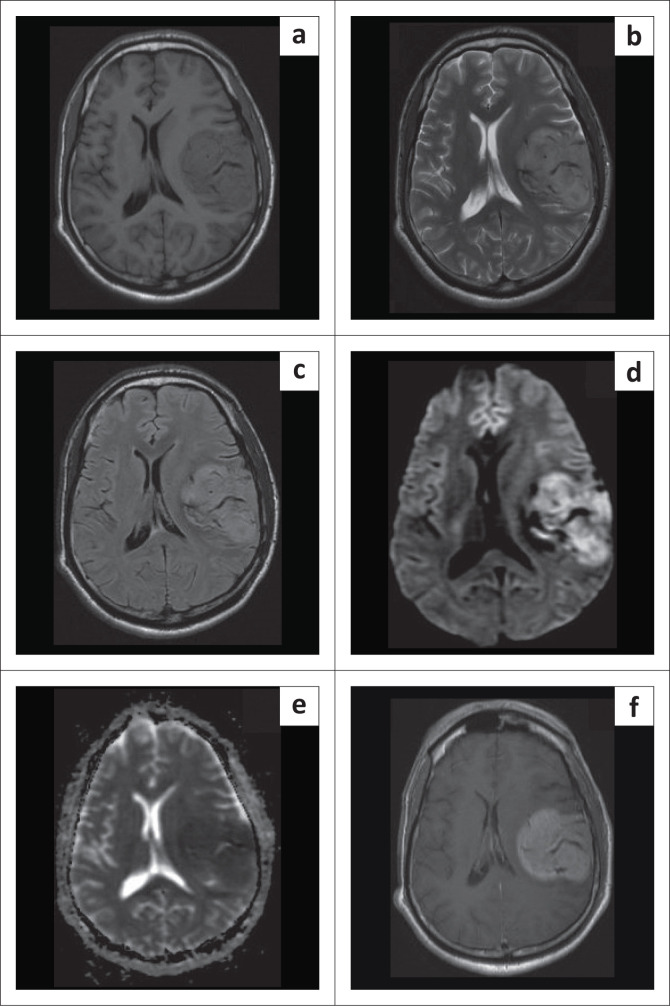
MRI brain shows a well-defined, lobulated, extra-axial lesion along left parietotemporal region. It appears isointense to grey matter on T1W (a) and T2W (b), and slightly hyperintense on fluid-attenuated inversion recovery (FLAIR) images (c). Multiple dilated vascular channels (flow voids) were seen within the lesion. It shows diffusion restriction on diffusion weighted imaging (DWI) (d) with low apparent diffusion coefficient (ADC) (e) values. It shows fairly homogeneous post-contrast enhancement (f).
